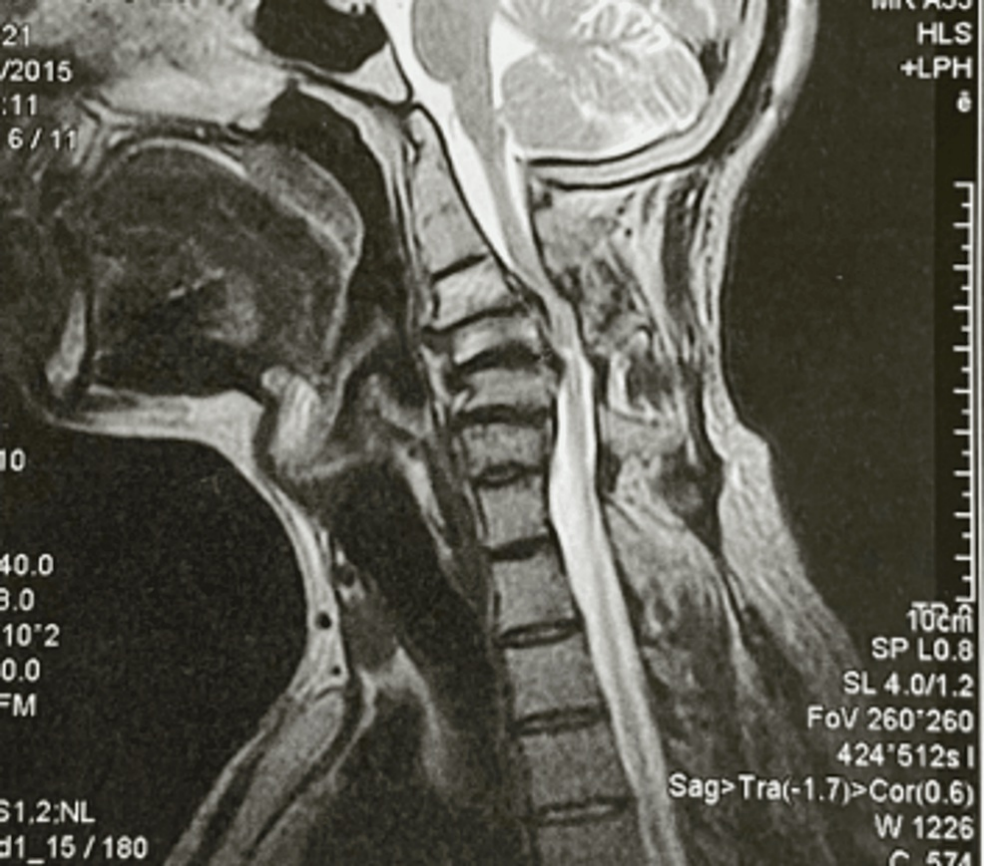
Preoperative MRI of the cervical spine with spinal cord compression present.
